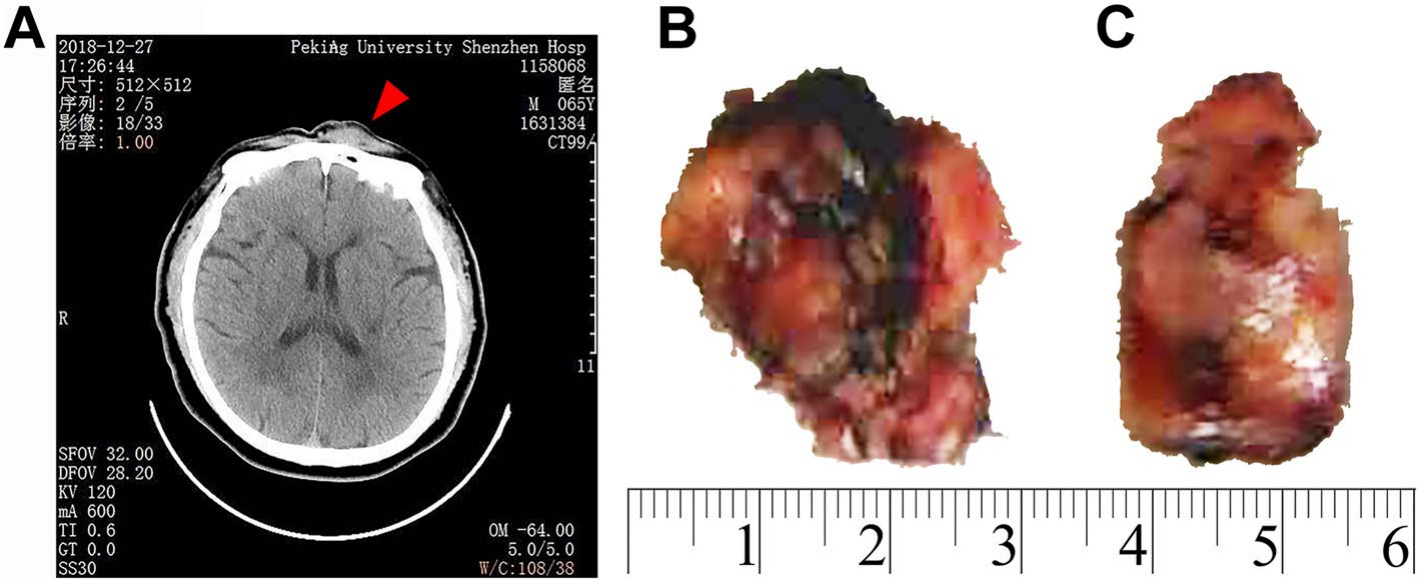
A Plain CT scan of the brain showed swelling of the subcutaneous soft tissue of the forehead with increased density and CT value of 63 HU. There was no invasion of the bone. B Grossly, the tumor was gray-red and 30 mm × 20 mm × 10 mm in size. C The cut surface of the mass was solid, grey-red, and slightly firm
